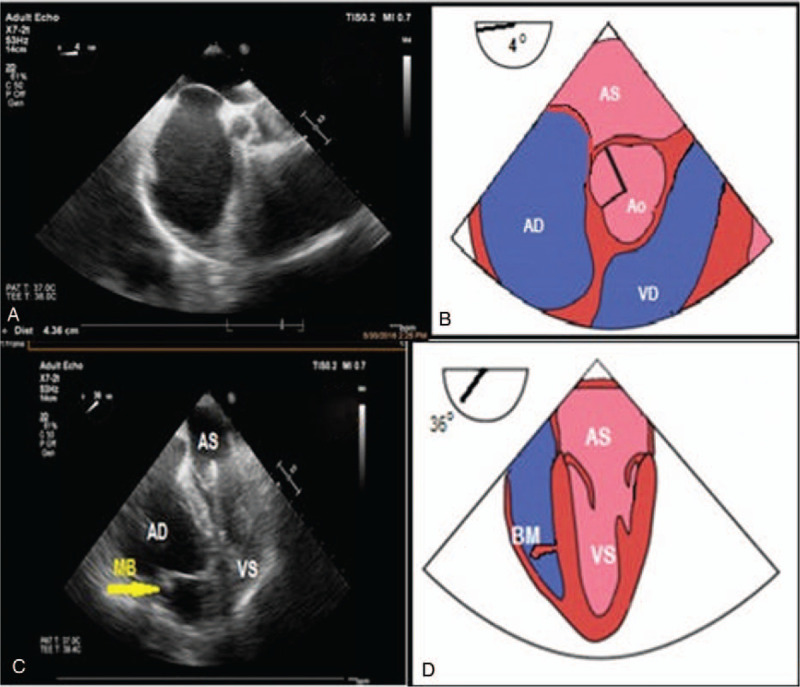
Transesophageal echography using a multiplanar probe. (A) Midesophageal view 4°: in the upper part of the image the left atrium can be seen- this is the closest anatomical structure to the esophagus. Separated by the interatrial septum, in the inferior part of the image lies the right atrium. The interatrial septum is convex towards the left atrium as the pressure inside the right atrium is high, given by the dilation of the right ventricle, tricuspid ring with subsequent tricuspid regurgitation. (B) Artwork with the structures seen in TEE. (C) Midesophageal view 36°: at the level of the right ventricle the moderator band (MB) can be visualized passing the ventricular cavity from the interventricular septum to the lateral wall where the anterior papillary muscle can be found. (D) Artwork with the moderator band as seen in TEE. TEE = transesophageal echocardiography.
